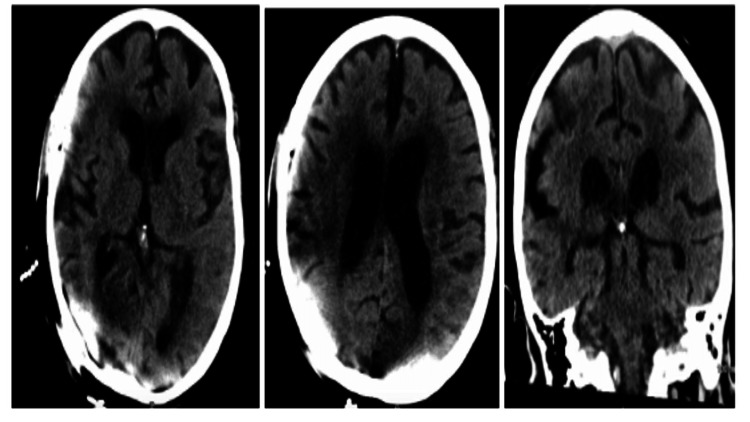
CT head without contrast showed no evidence of an acute abnormality contributing to the patient’s encephalopathy
